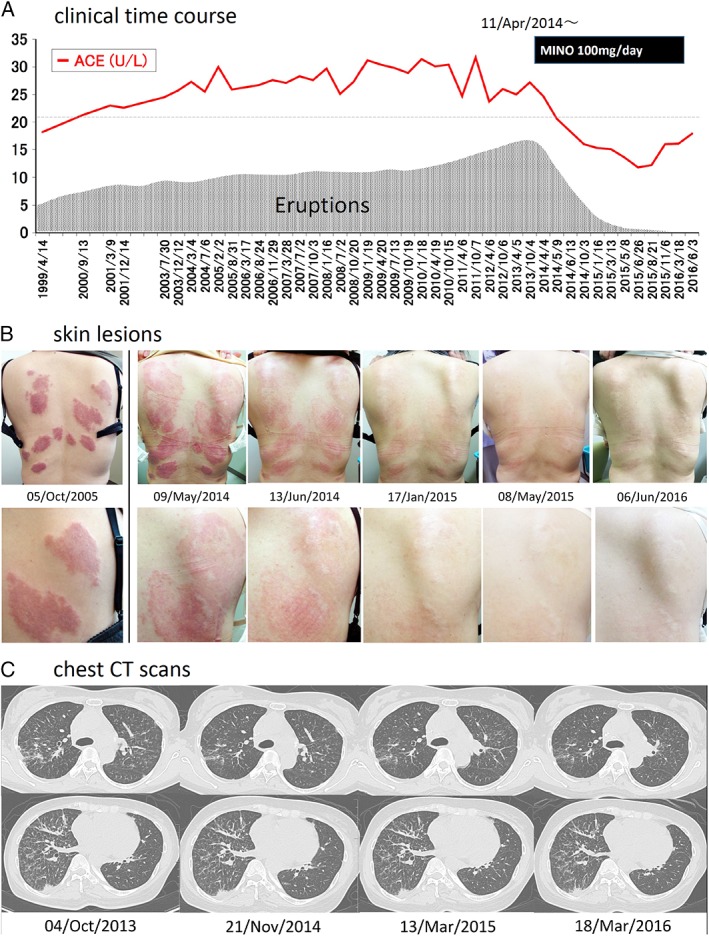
(A) Clinical time course. Oral administration of minocycline was started at 100 mg/day on 11 April 2014. After treatment initiation, the angiotensin‐converting enzyme levels, which had remained high since 2000, rapidly decreased to the normal range. (B) Time course of skin sarcoidosis. Since the early stage of the disease, multiple red sarcoid eruptions appeared mainly on the skin of the back. After minocycline monotherapy initiation, these eruptions gradually resolved and only slight scars remained after approximately 1 year of therapy. (C) Chest computed tomography (CT). High‐resolution chest computed tomography revealed small nodular lesions, reticular lesions, and consolidations along the interstitium, including the bronchovascular bundle or interlobular septa. Although some lesions resolved after the start of minocycline monotherapy, improvement in these lesions was less as compared with those for skin eruptions.
